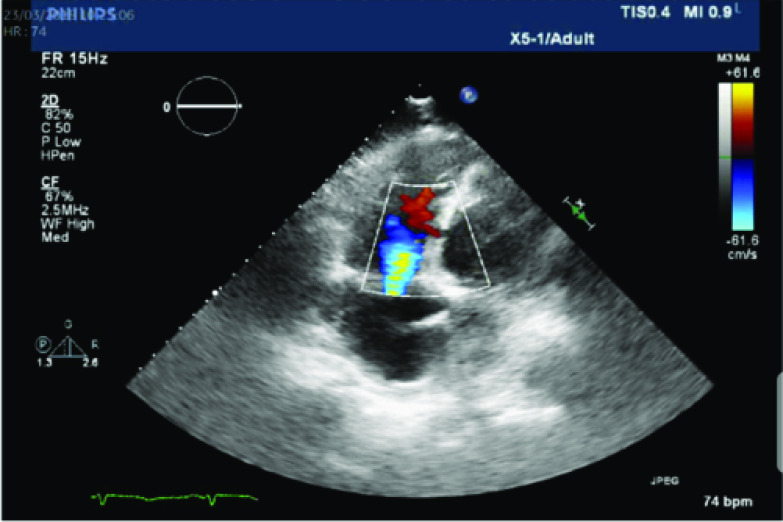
A large posterobasal ventricular septal defect as seen on transthoracic echocardiography.
